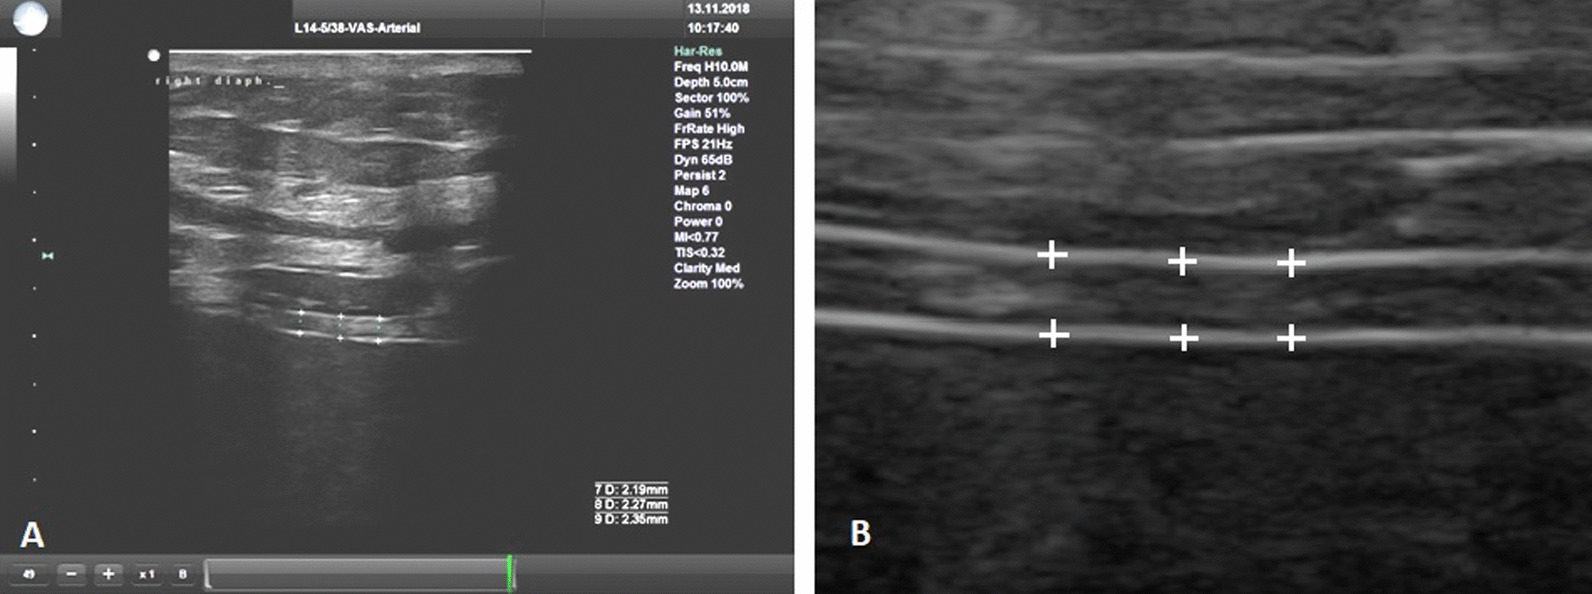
Ultrasound measurement of the right side of the diaphragm, linear probe, 10 MHz (a), measurement was made from the middle of the pleural line to the middle of the peritoneal line (b) detail
